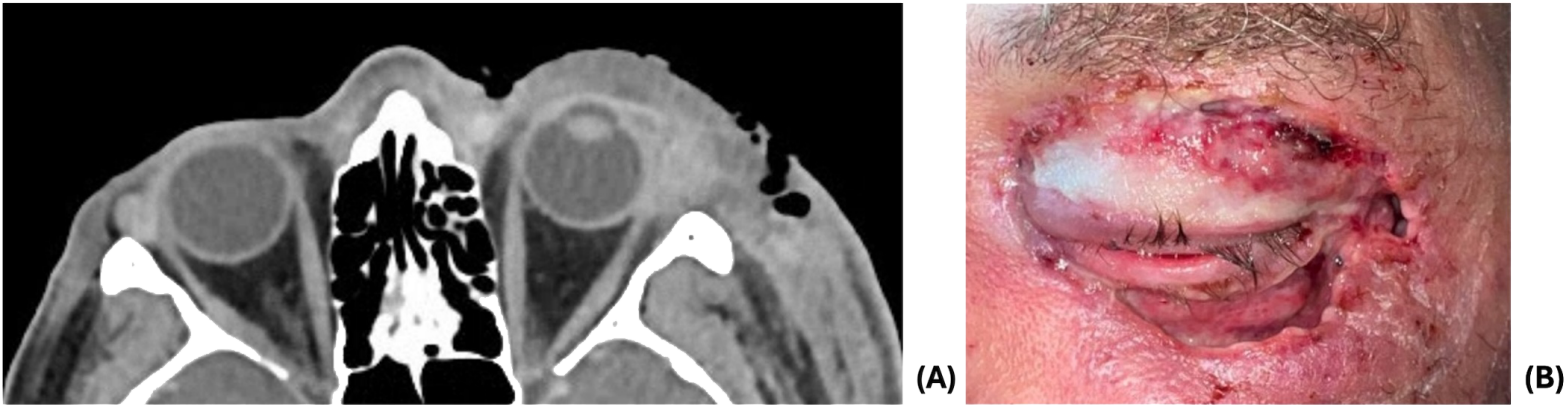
(A) CT demonstrating increased left periorbital soft tissue swelling extending to left-sided face and presence of gas within thickened skin and soft tissues. (B) 1 day post-debridement.
